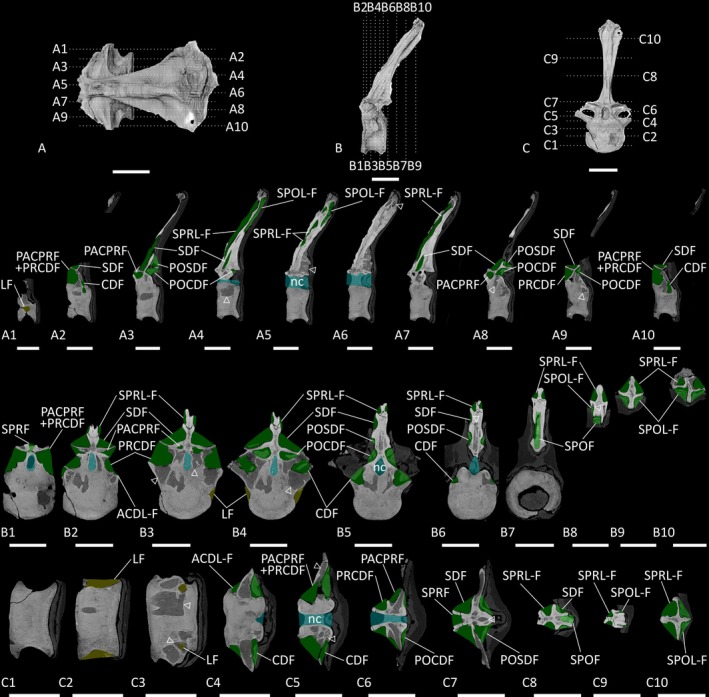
CT scan of Rebbachisauridae indet. anterior caudal vertebra MDPA‐Pv 007. (A–C) 3D rendering (A) dorsal view, (B) left lateral view, (C) anterior view; (A1–A10) CT scan slices in parasagittal cross‐section, (B1–B10) CT scan slices in transverse cross‐section, (C1–C10) CT scan slices in horizontal cross‐section. ACDL‐F, anterior centrodiapophyseal lamina‐fossa; CDF, centrodiapophyseal fossa; LF, lateral fossa; nc, neural canal; PACPRF, parapophyseal centroprezygapophyseal fossa; POCDF, postzygapophyseal centrodiapophyseal fossa; poz, postzygapophysis; POSDF, postzygapophyseal spinodiapophyseal fossa; PRCDF, prezygapophyseal centrodiapophyseal fossa; prz, prezygapophysis; SDF, spinodiapophyseal fossa; SPOF, spinopostzygapophyseal fossa; SPOL‐F, spinopostzygapophyseal lamina‐fossa; SPRF, spinoprezygapophyseal fossa; SPRL‐F, spinoprezygapophyseal lamina‐fossa; tp, transverse process. Key: Blue highlight, neural canal; green highlight, neural spine fossa; yellow highlight, centrum fossa; white arrow, pneumatic bone texture. Scale bars = 100 mm.
